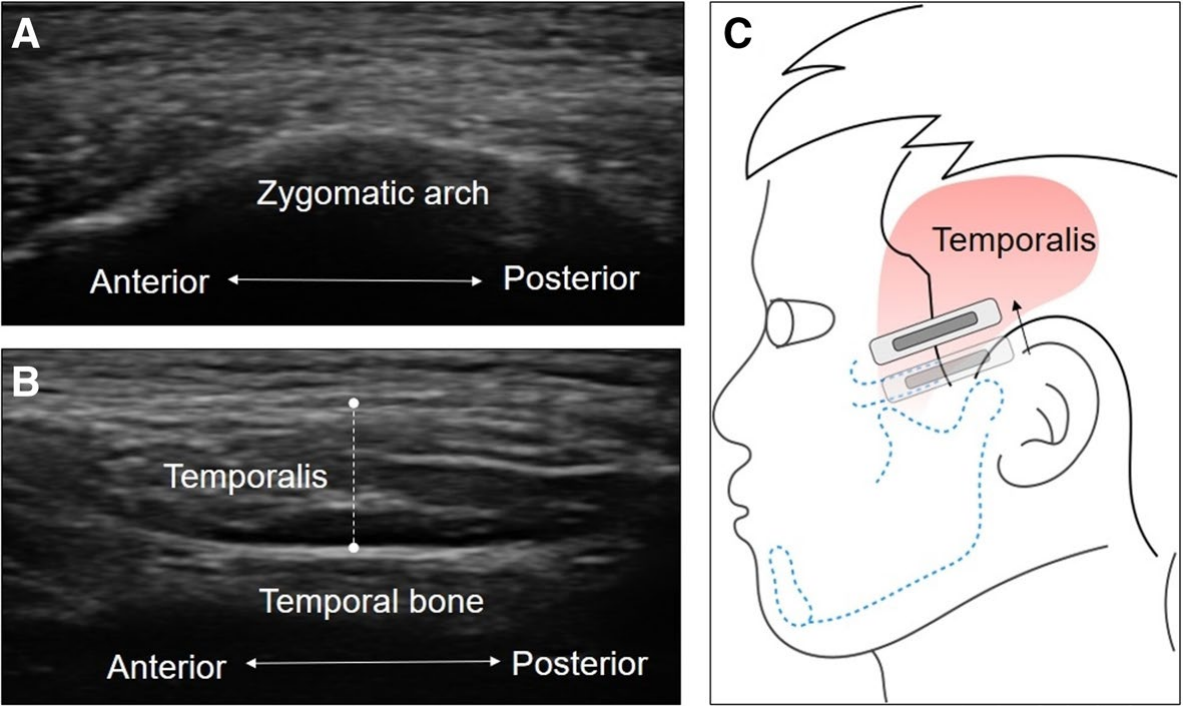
Ultrasound imaging of the zygomatic arch (A) and the temporalis muscle (B) as the transducer is moved towards the temporal fossa (C). Note that the length of the dashed lines indicates the thickness of the muscle.
